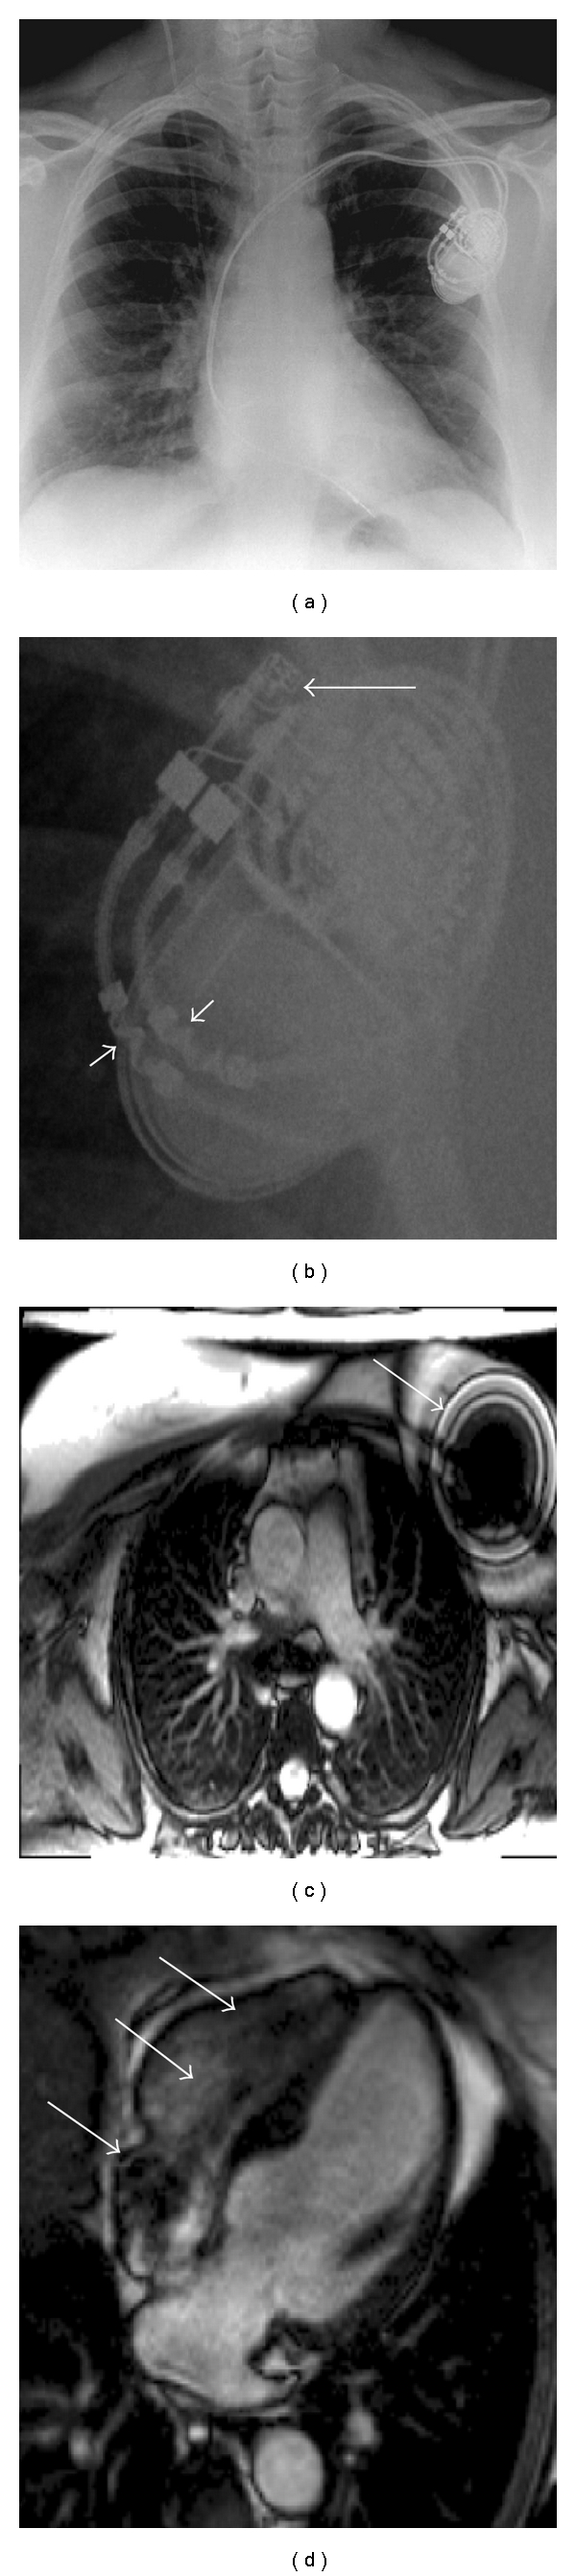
A CMR conditional pacemaker. (a, b) show the pacemaker on a chest X-ray. On (b) the arrows point to MRI conditional markers on the header and leads. (c) shows a large artifact from the pacemaker box on a transverse white blood stack. (d) shows suseptibility artifact from the pacemaker leads in the heart.
